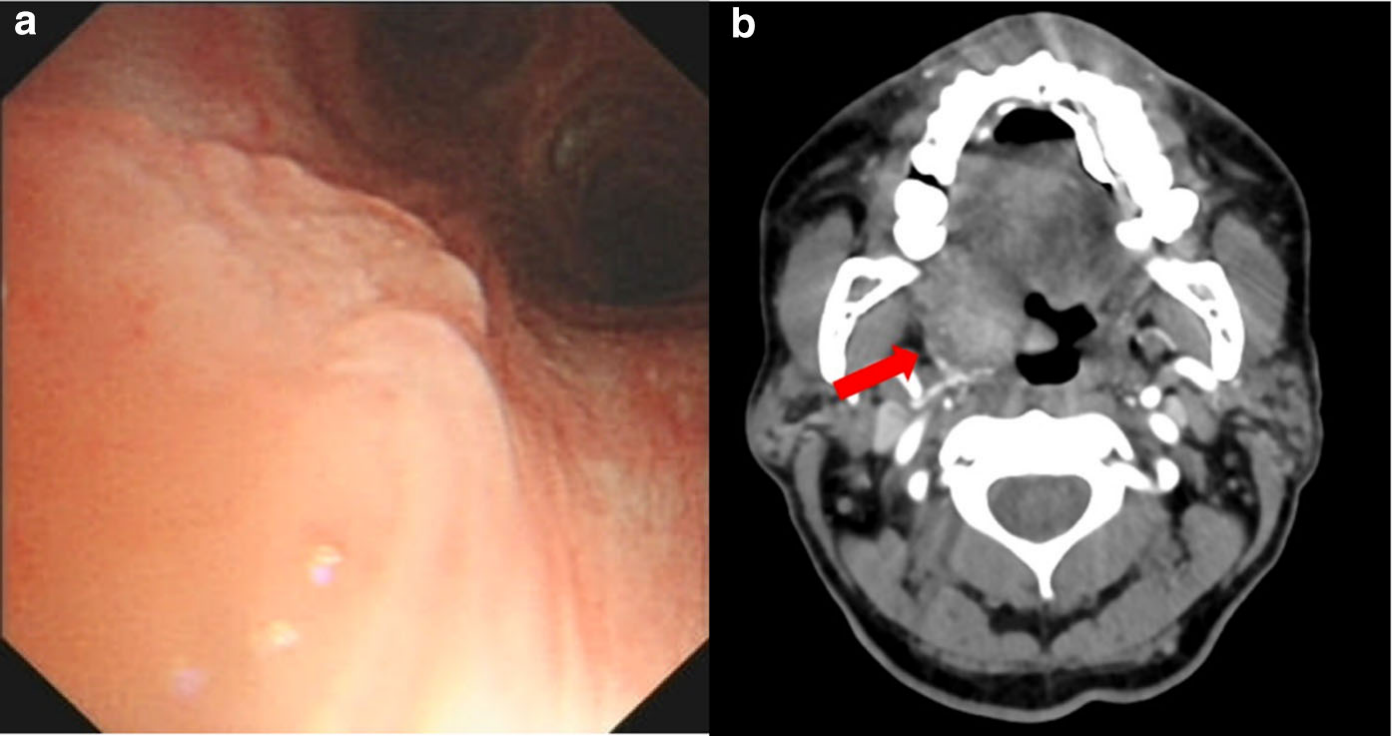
Upper respiratory tract manifestations of talaromycosis. a Bronchoscopy demonstrating irregular bronchial mucosal surface, in a 34-year-old immunocompromised female with a STAT3 mutation. Tm was grown from biopsied tissue [59]. b Computed tomography (CT) angiogram of the neck demonstrating an ill-defined mass along the right lateral aspect of the hypopharynx involving the base of the tongue, right lingual tonsil, and right vallecula extending along the right palatine tonsil and into the pharyngeal space, in a 63 year-old man with HIV [27]
